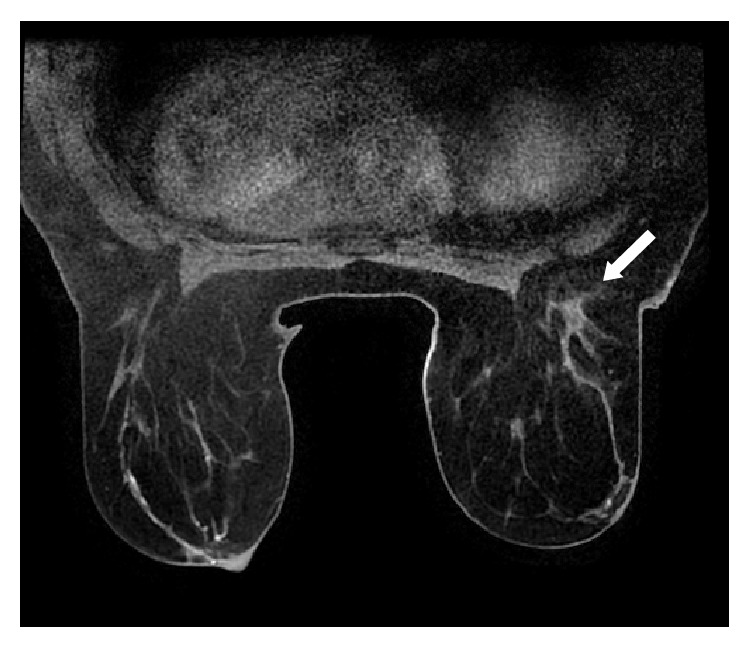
Contrast enhanced dynamic MRI of breast. The patient had surgical excision for granulomatous mastitis. Postcontrast enhancement is still seen in operative bed long time after surgery due to fibrosis in this region. It showed progressive enhancement on dynamic curve (not shown here).
